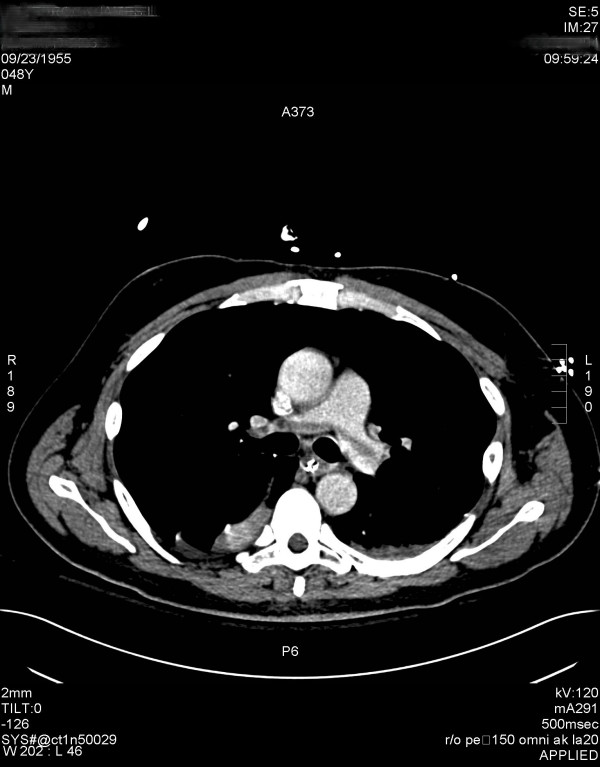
CT Angiogram obtained after 78 hours of infusion of rhAPC demonstrating a large saddle pulmonary embolism.
